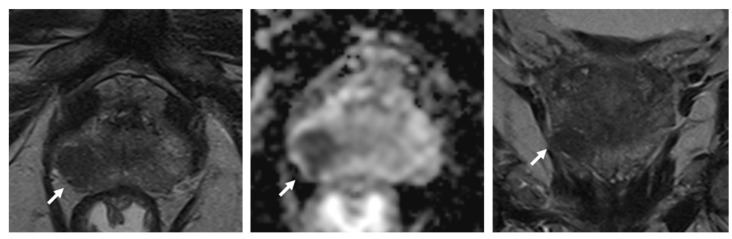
76-year old patient with elevated PSA value and a suspicious lesion (white arrows) in the right peripheral zone. In this case, the extracapsular tumor extension is visualized best on the coronal T2-weighted sequence.
